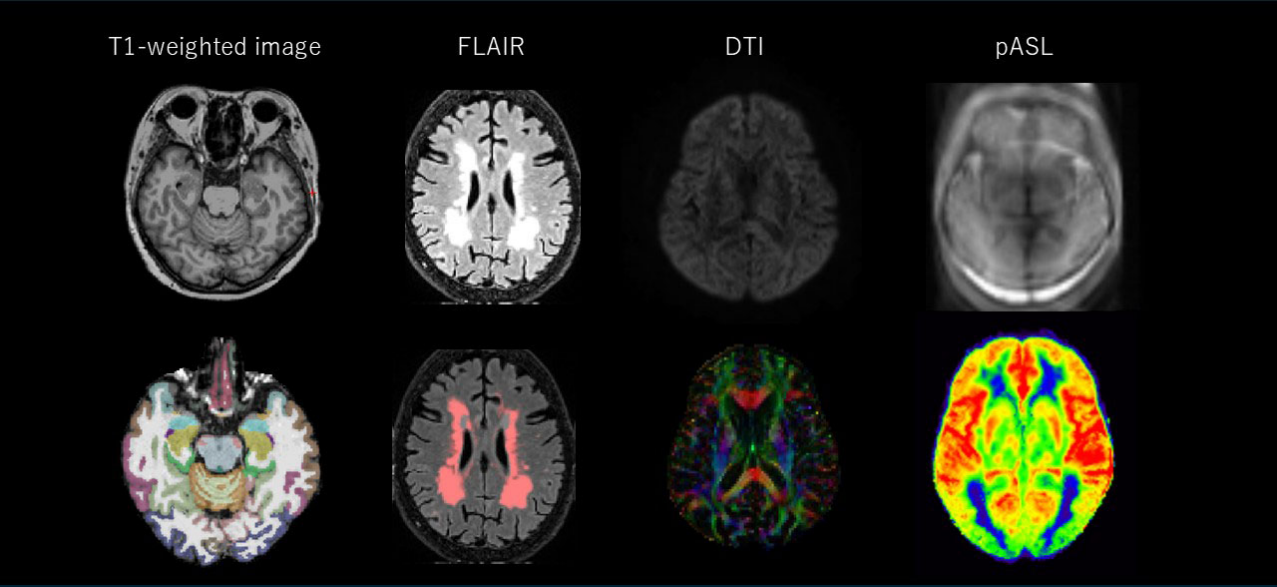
Representative MRI images. MRI images in the upper row are unprocessed images, while those in the lower row are processed images. The DTI color map shows the direction of the projection fibers (*z*-axis, blue), the association fibers (*y*-axis, green), and the subcortical fibers (*x*-axis, red). FLAIR, fluid-attenuated inversion recovery; DTI, diffusion tensor imaging; pASL, pulsed arterial spin labeling.
